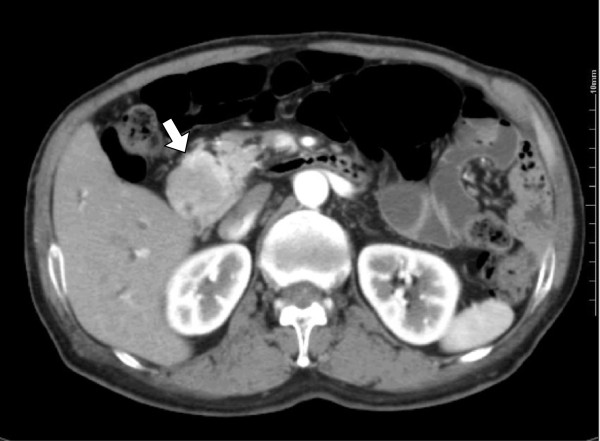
Findings of an abdominal contrast-enhanced computed tomography scan. The scan demonstrates a 30-mm tumor in the head of the pancreas (*arrow*), with slow enhancement.
